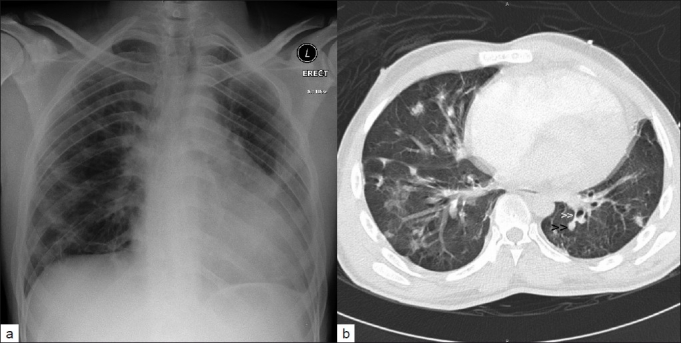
(a) Chest X-ray showing cardiomegaly with bilateral lung infiltrates and (b) CT scan of chest showing bilateral irregular pulmonary infiltrates with multiple nodules (black arrowheads) with distinct central feeding vessel (white arrowheads), consistent with septic pulmonary emboli in a patient with intravenous drug abuse and tricuspid valve endocarditis
